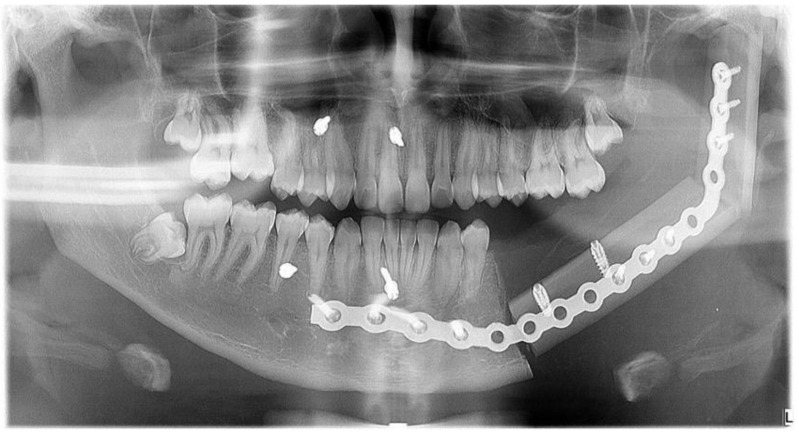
Panoramic radiographic, immediate postoperative.
